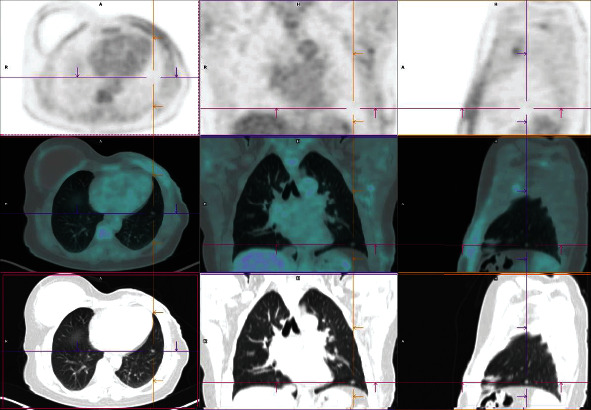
PET/CT images showing an increased metabolic activity in the pulmonary suspected lesion.
